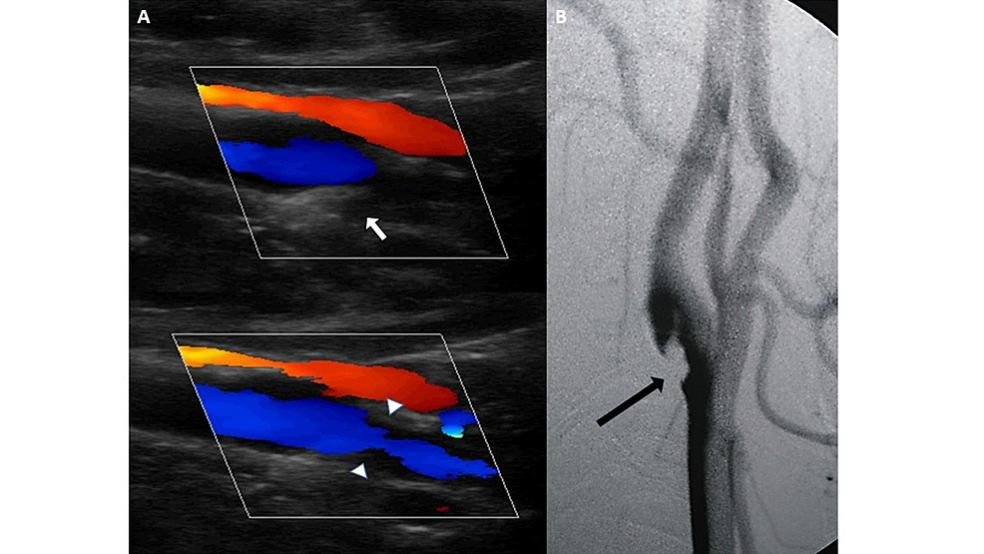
Carotid web (A) Ultrasound carotid duplex shows intimal thickening greater than 0.9 mm and the presence of an isoechoic membrane of 0.75 cm x 0.25 cm at the level of the left internal carotid artery that produces 50% narrowing (arrow) and turbulent flow (arrowhead) with increased systolic velocity of 137 m/s. (B) In digital subtraction angiography, a shelf-shaped filling defect is observed at the level of the posterior wall of the left carotid bulb (arrow) that extends to its ascending cervical portion. (C1).
